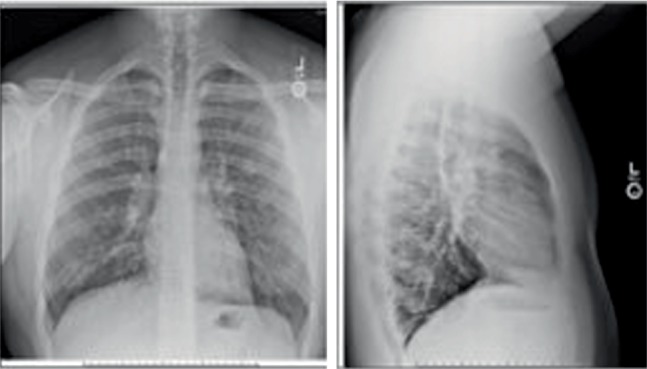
Two-view chest X-ray: increased pulmonary markings and possible multi-lobar pneumonia.
